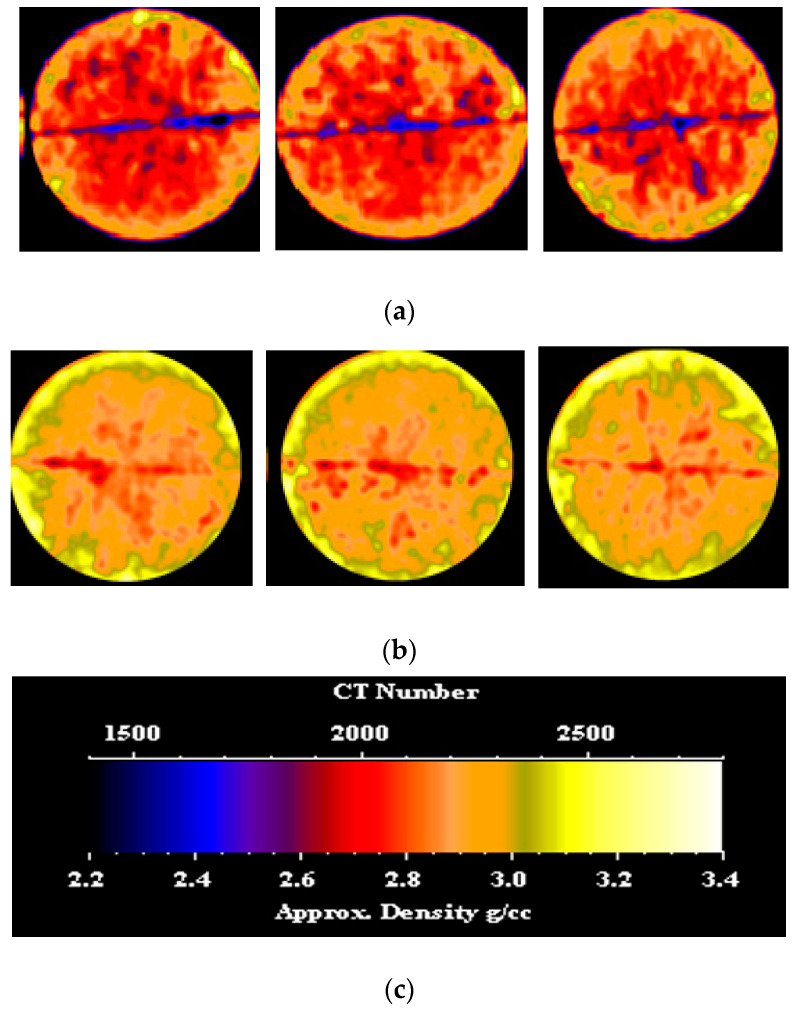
(a) CT scan of the fractured sample before PAM/PEI gel treatment; (b) CT scan of the fractured sample after PAM/PEI gel treatment; (c) color code scale.
